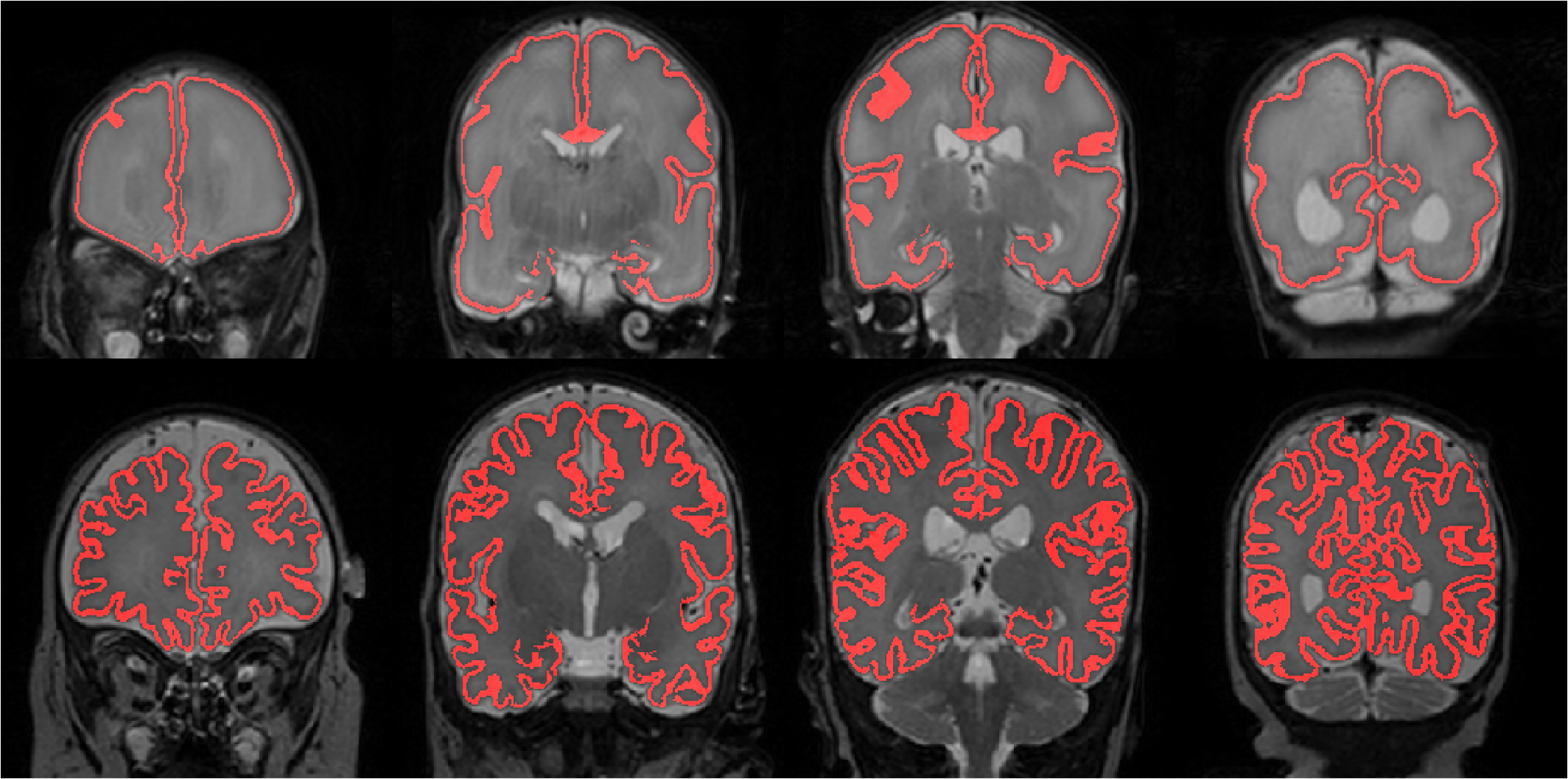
Automatic segmentation of CoGM at 30 (top) and 40 weeks PMA (bottom) for the same patient, shown in four slices of the T2-weighted images. Note that, because of the limited resolution, CSF inside the sulci was not always visible, which resulted in local overestimation of cortical thickness.
